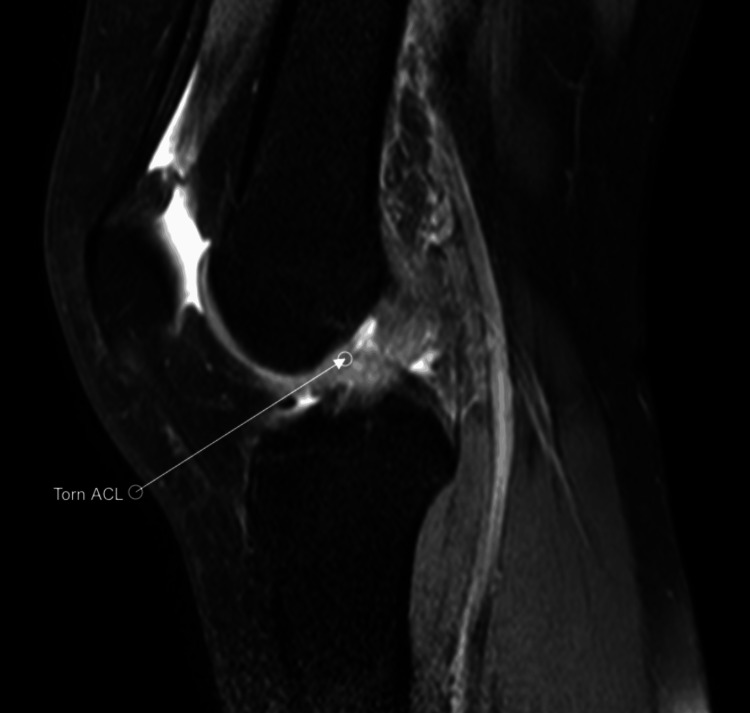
T2 MRI sagittal view of the left knee showing torn ACL ACL: Anterior cruciate ligament.
